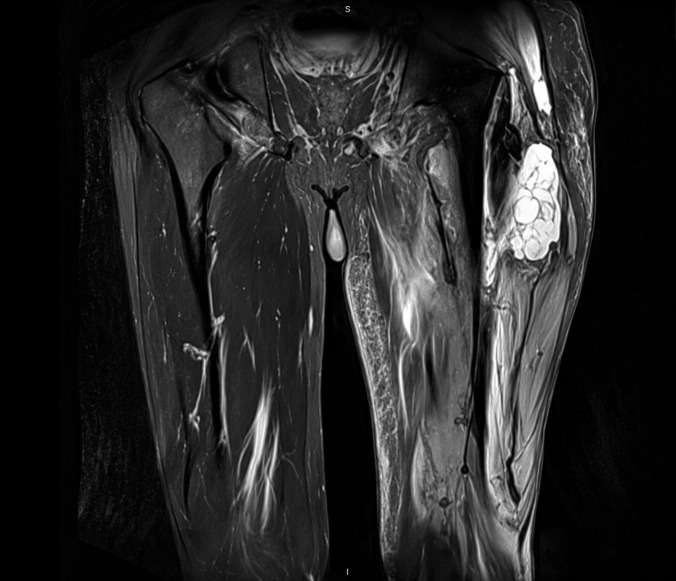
MRI Pelvis coronal arrow pointing to growth of left femur metastasis.
